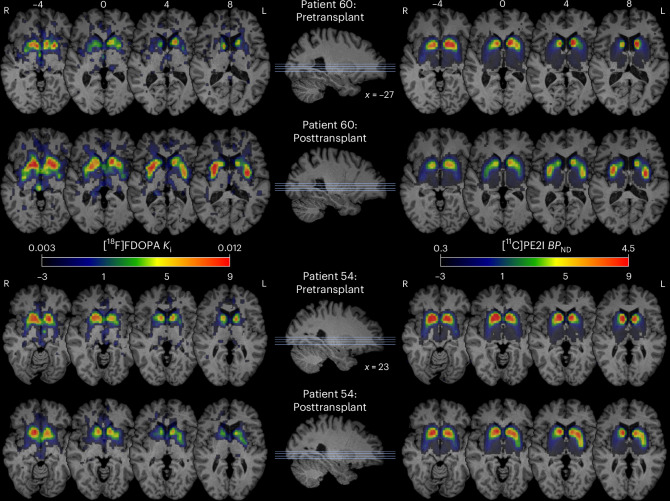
Dopaminergic PET after hfVM transplantation. Representative [^18^F]FDOPA (left) and [^11^C]PE2I (right) parametric images for two bilaterally transplanted patients whose postoperative levels of dopamine synthesis and transporter expression were either high (patient 60) or comparatively modest (patient 54). Dopaminergic PET data are overlaid onto patient T1-weighted magnetization prepared rapid gradient echo (MPRAGE) scans rigidly aligned to the Montreal Neurological Institute (MNI) template and displayed as axial slices covering the putamen; R, right; L, left.
